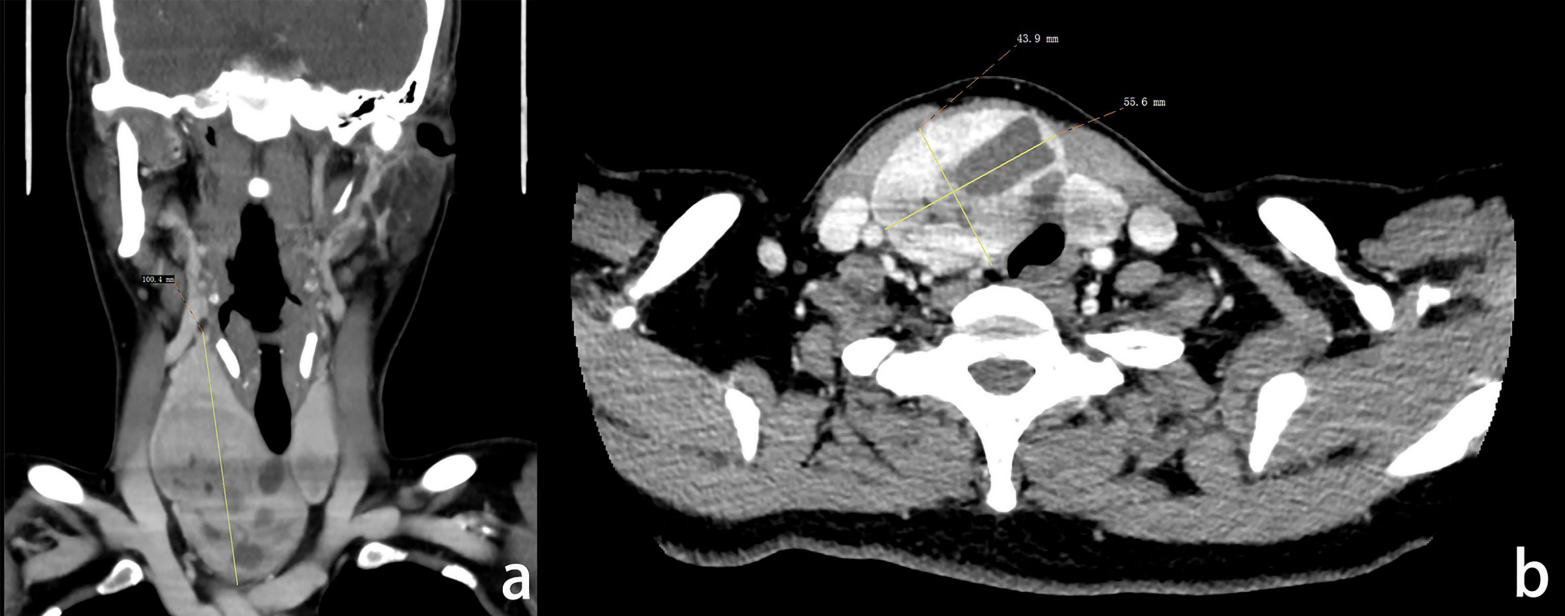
(A) The coronal image of enhanced CT of the patient’s neck, the maximum diameter of the gland was 100.4 mm; (B) The horizontal image of enhanced CT of the patient’s neck, the maximum diameter of tumor cross-section was 55.6×43.9 mm.
